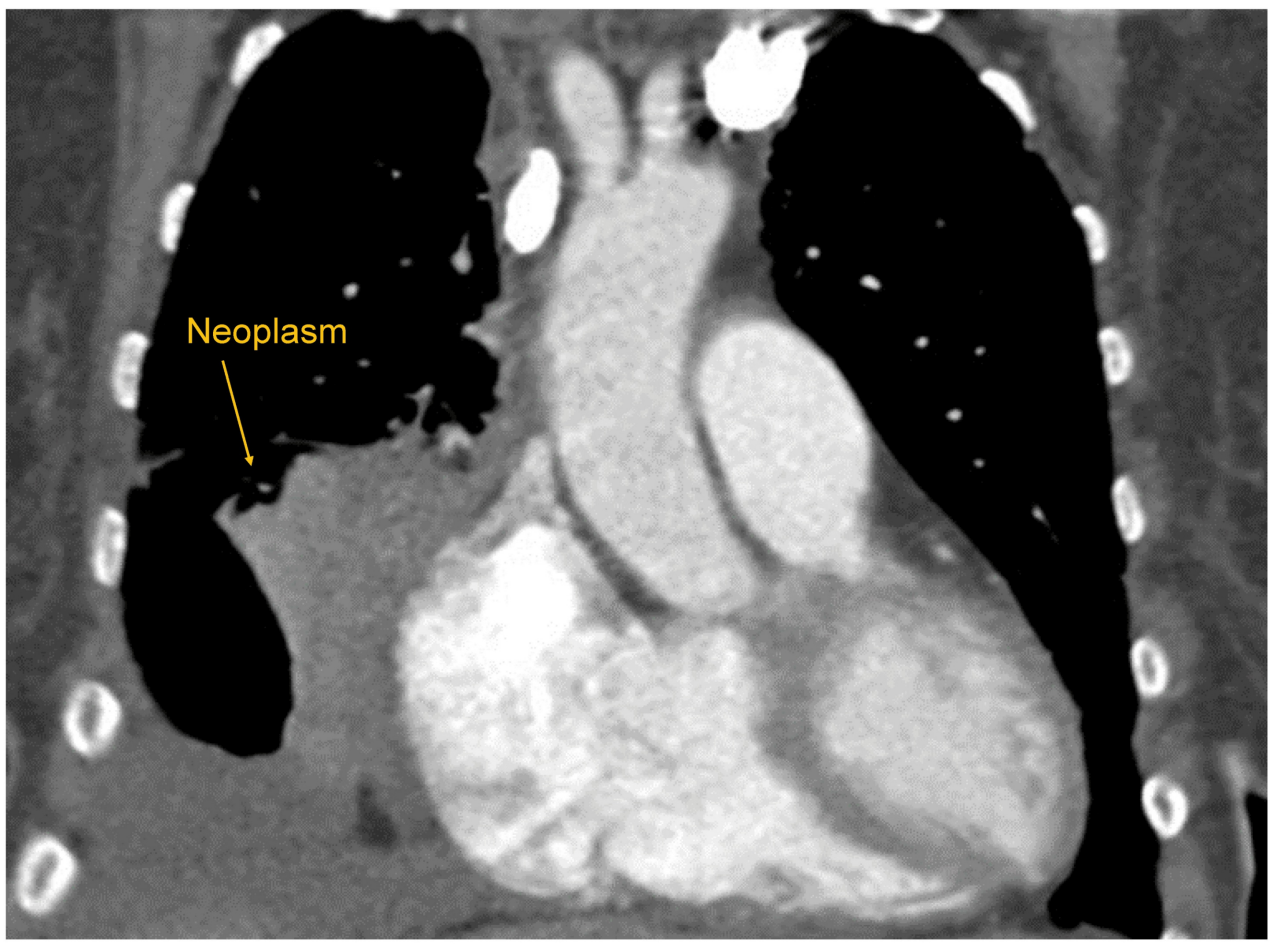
Coronal view of chest CT of a 51-year-old with dyspnea and cough, showing aggressive right-sided lung neoplasm (Case 1)
